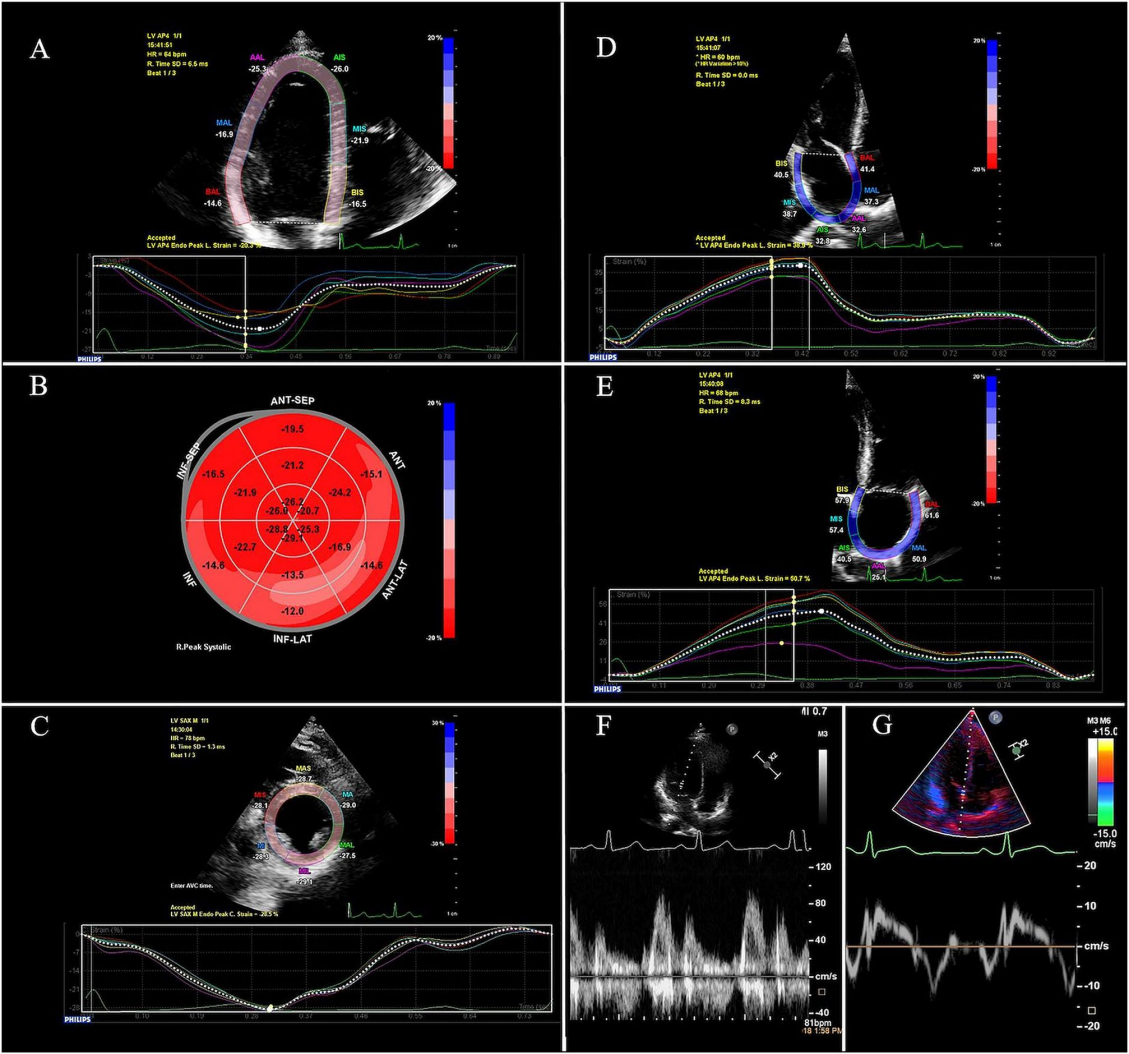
28-year-old male patient, with a 15-year history of T1DM. His HbA1c was 6.5% at inclusion. No signs of hypertensive heart disease. (A) Apical four-chamber view depicting the region of interest and the segmental longitudinal strain curves created by the speckle-tracking software. (B) Bull’s eye demonstrating the segmental peak systolic longitudinal strain values in the entire LV. Value of GLS is − 20.4%. (C) LV parasternal short-axis view at mid-papillary level depicting the region of interest and the segmental circumferential strain curves created by the software. Value of GCS is − 28.5%. (D) LA-focused apical four-chamber view depicting the region of interest and the LA strain curve created by the speckle-tracking software. LA reservoir strain: 38.9% (E) RA-focused apical four-chamber view depicting the region of interest and the RA strain curve created by the speckle-tracking software. RA reservoir strain: 50.7%. (F) Spectral Doppler curve of the mitral inflow. Mitral E: 81 cm/s, Mitral A: 62 cm/s. (G) Pulsed tissue Doppler curve measured on the septal border of the mitral annulus. Mitral e′: 11.3 cm/s.
